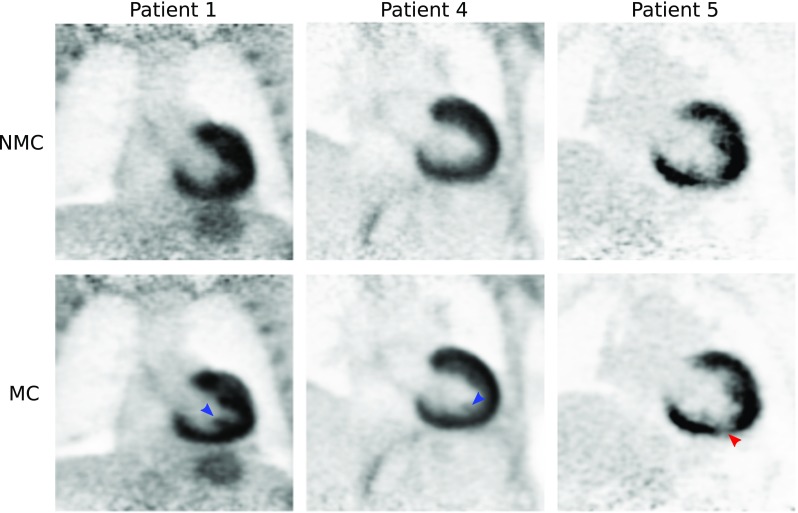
Coronal slice for three representative patients (*columns*) showing uncorrected (NMC) and motion-corrected (MC) viability ^18^F-FDG PET images. Improvements in image quality can be observed when applying MC, particularly in small structures (*blue and red arrows*), compared to NMC
